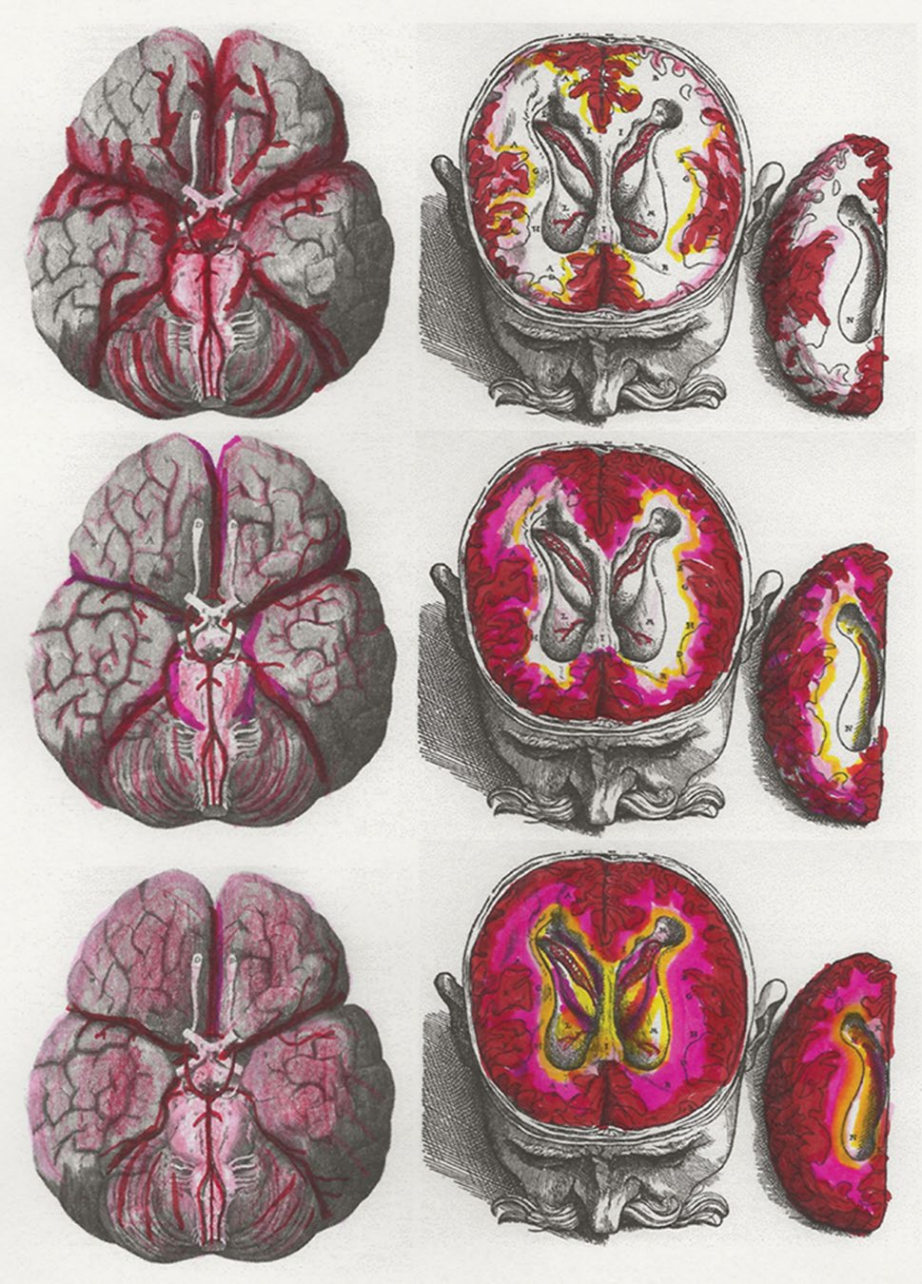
Cerebrospinal fluid (CSF) tracers have a brain-wide distribution along the subarachnoid space and perivascular spaces (Virchow–Robin spaces) through the glymphatic pathway [2]. Dandy used India ink as a CSF tracer to show this rapid redistribution from the basal cisterns into the subarachnoid space adjacent to the circle of Willis and along its major arterial branches (top row). The glymphatic pathway was recently confirmed in humans by observing the distribution of a gadolinium CSF tracer (gadobutrol) from the subarachnoid space deep into the brain parenchyma using serial magnetic resonance imaging (23). The gadobutrol CSF tracer enters the cortical mantle by 6–12 h (middle row). By 24–48 h, it is found deep within brain tissue (bottom row). The intrathecal gadobutrol is completely cleared at 4 weeks. The red, pink, yellow, and white color scale qualitatively indicates tracer enrichment. Adapted from Ringstad et al. [23] by using the 1664 engravings in *Cerebri Anatome* by Sir Thomas Willis (left column) and the 1555 engravings in *De Humani Corporis Fabrica* by Andreas Vesalius (middle and right columns). We encourage readers to visit the link to the supplemental video kindly provided by Ringstad and colleagues (https://www.ncbi.nlm.nih.gov/pmc/articles/PMC6124518/bin/jciinsight-3-121537-s253.mp4)
